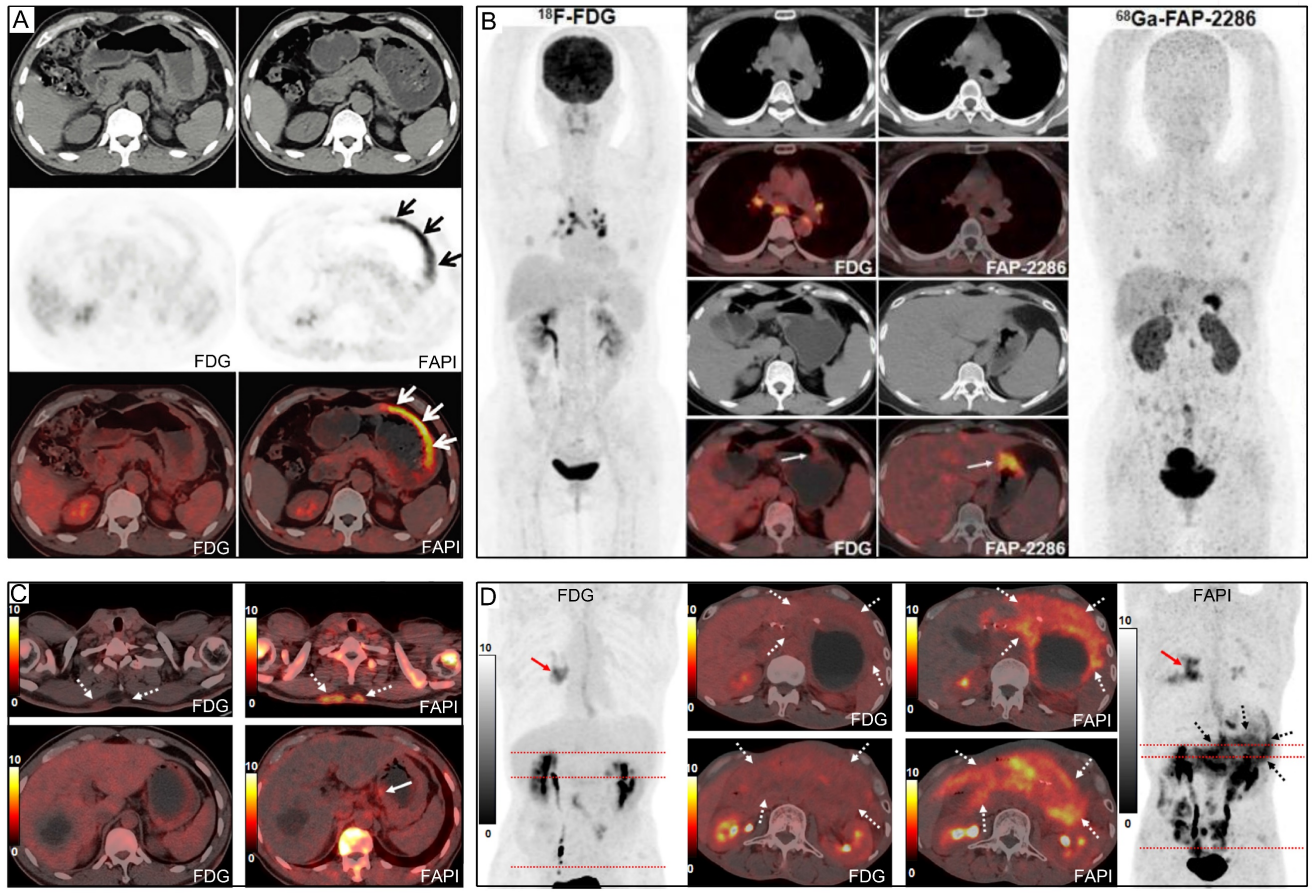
Representative ^18^F-FDG and ^68^Ga-FAPI PET/CT images of primary and metastatic gastric signet ring cell carcinoma (GSRCC). (A) A 55-year-old man with GSRCC underwent ^18^F-FDG-PET/CT for initial staging. ^18^F-FDG PET/CT showed normal findings, while ^68^Ga-FAPI PET/CT revealed intense uptake along the gastric wall. (B) A 40-year-old woman with GSRCC underwent PET/CT for tumour staging. ^68^Ga-FAP-2286 showed higher uptake in the primary tumour than ^18^F-FDG. (C) A 52-year-old man with widespread subcutaneous and bone metastases underwent ^18^F-FDG PET/CT for localizing the primary tumour. However, no intense ^18^F-FDG uptake that likely presenting the primary tumour was observed. ^68^Ga-FAPI PET/CT revealed intense uptake in the lesser curvature of the stomach. A subsequent gastroscopic biopsy confirmed the diagnosis of GSRCC. (D) A 49-year-old man with prior gastrectomy for GSRCC presented with progressive abdominal pain. ^68^Ga-FAPI PET/CT revealed higher radiotracer uptake and larger disease extent than ^18^F-FDG in peritoneal metastases. Source: From Pang, Yizhen *et al.* (2021), Pang, Yizhen *et al.* (2023), and Chen, Haojun *et al.* (2023) with modifications.
